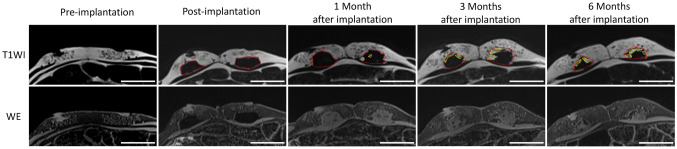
Magnetic resonance imaging evaluation of the volume of the implant aggregate and the newly formed adipose tissue. The red dotted lines indicate the area of the implant aggregate, and the yellow dotted lines indicate the area of newly-formed adipose tissue. The areas of the implant aggregates were confirmed at all time points. The newly formed adipose tissue was identified as hyperintense in the T1-weighted images and the hypointense in water excitation images at 1, 3, and 6 months after implantation. Scale bar: 5 cm
